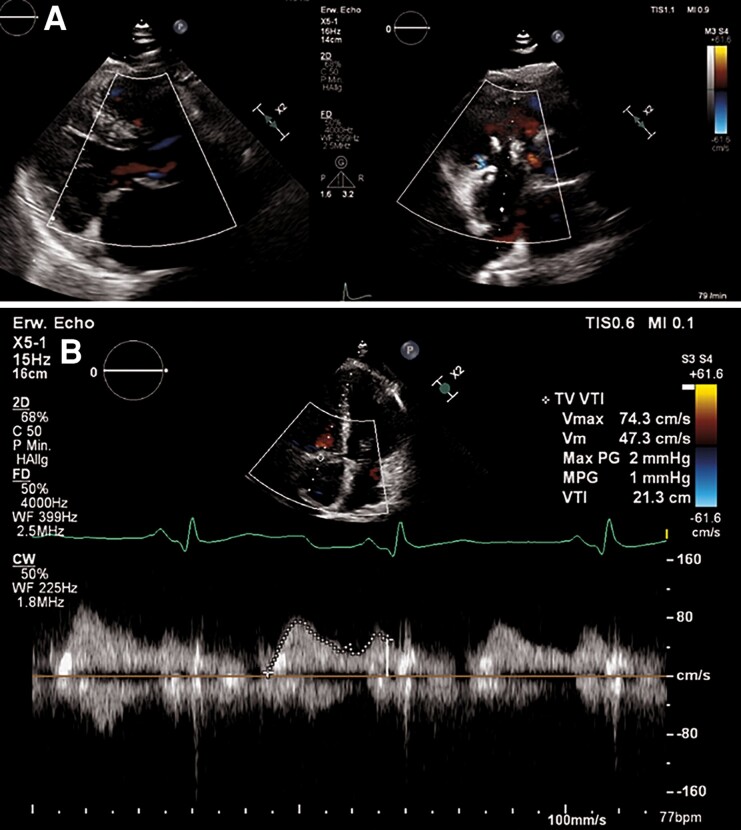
Pre-discharge transthoracic echocardiography with tricuspid valve repair. (*A*) Evidence of normal coaptation at the level of tricuspid valve annulus. (*B*) Trivial tricuspid valve regurgitation with mean pressure gradient 1 mmHg.
